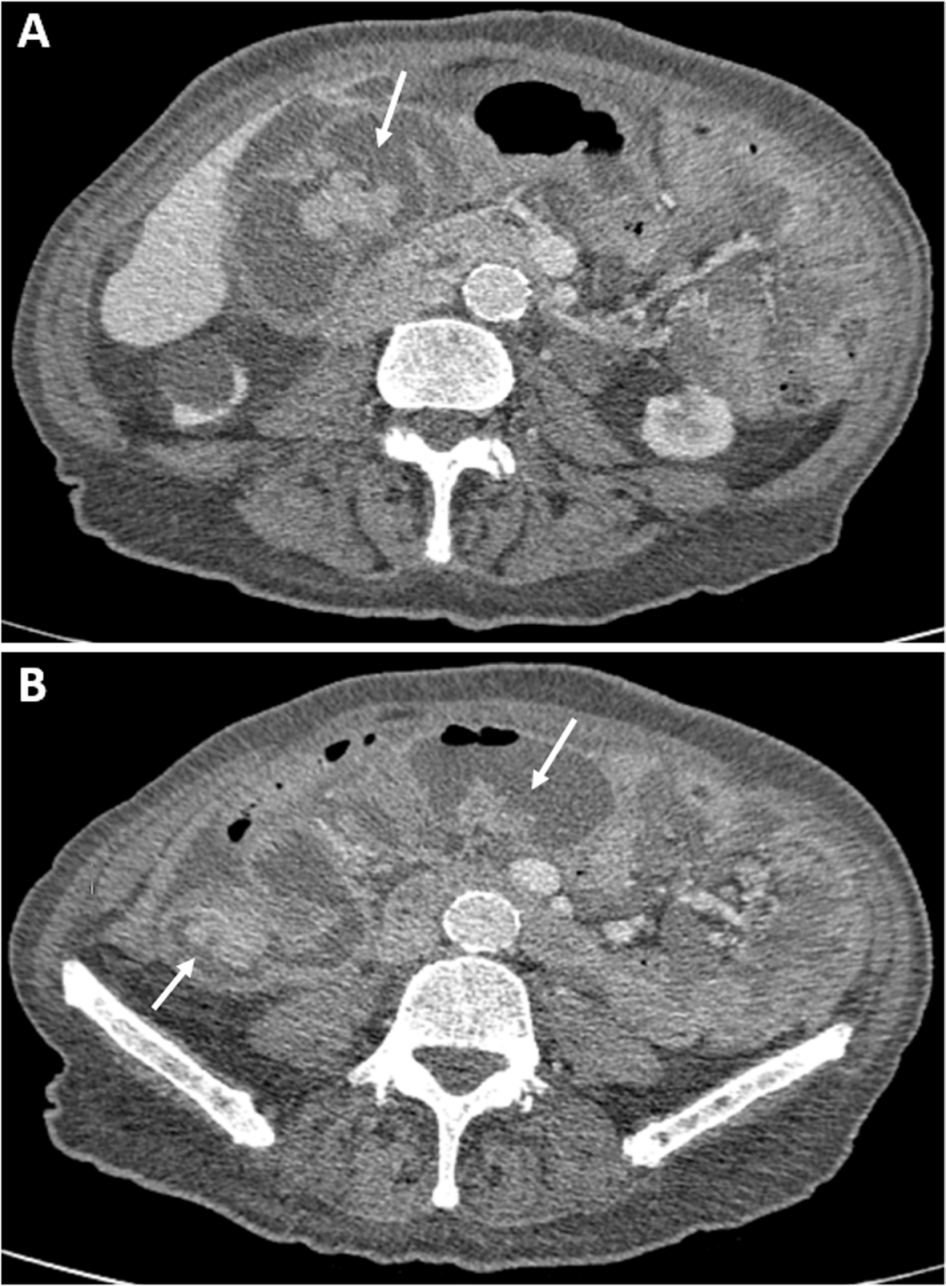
Abdominal CT scan of recurrent disease. Cross-sectional images showing dilation of multiple loops of the small bowel and intraluminal mucosal polypoid lesions with contrast enhancement, indicated by the white arrows
